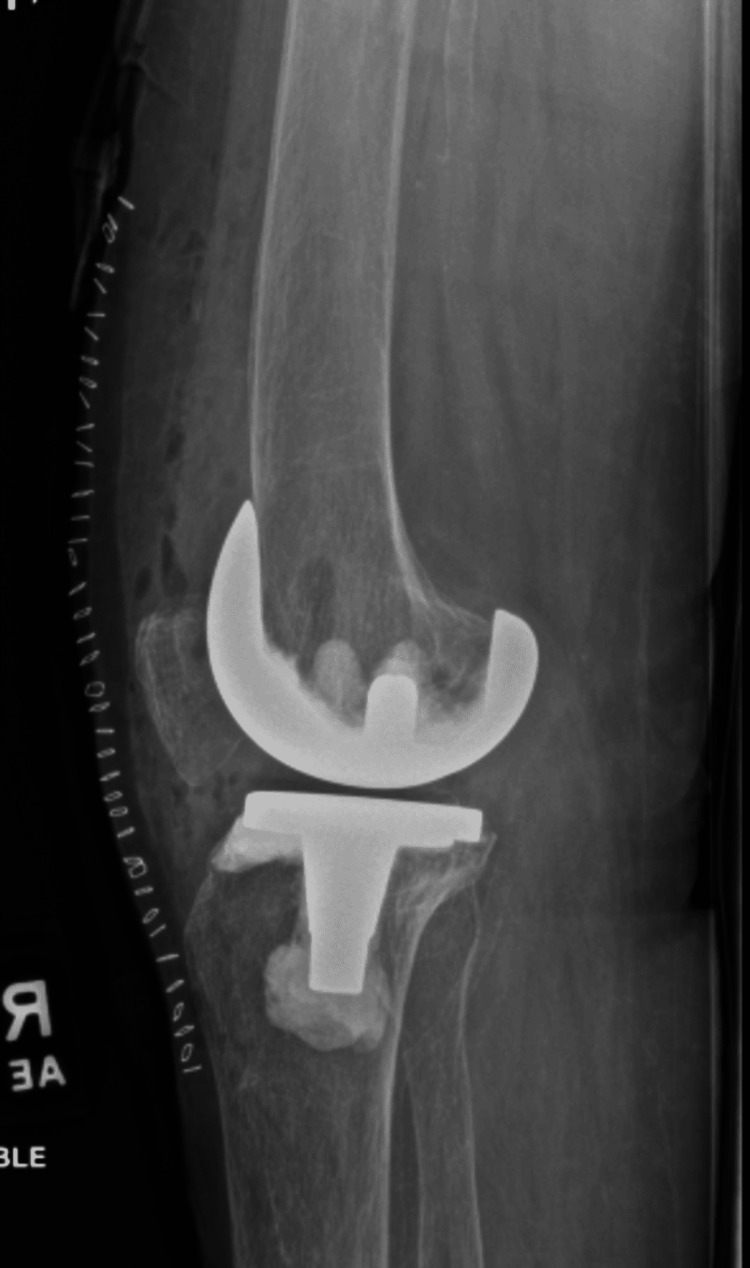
Postoperative plain radiography, lateral, non-weight bearing view, demonstrating new prosthesis.
